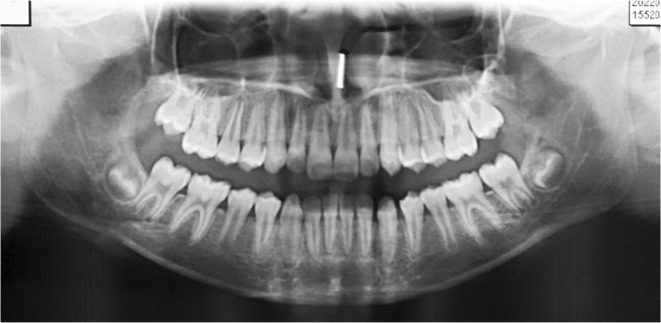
The orthopantomogram of the patient.
